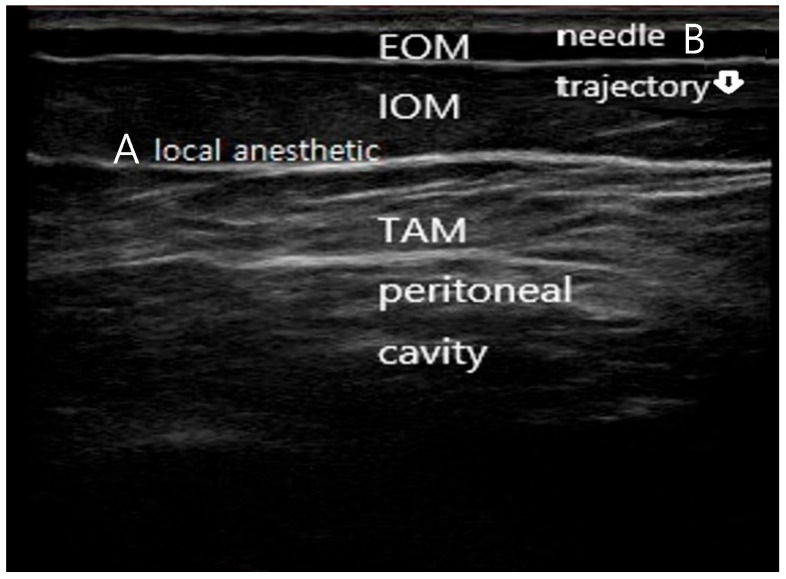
Ultrasound-guided subcostal transversus abdominis plane (TAP) block technique. (A) Ultrasound image demonstrating the subcostal abdominal wall anatomy, including the external oblique muscle (EOM), internal oblique muscle (IOM), transversus abdominis muscle (TAM), and peritoneal cavity. The target fascial plane between the internal oblique and transversus abdominis muscles is indicated. (B) In-plane needle advancement with a near-vertical trajectory toward the subcostal TAP plane under real-time ultrasound guidance, with hydrodissection confirming correct deposition of local anesthetic within the target fascial plane. The needle is faintly visualized advancing in-plane toward the target fascial plane, consistent with real-time ultrasound-guided subcostal TAP block technique.
